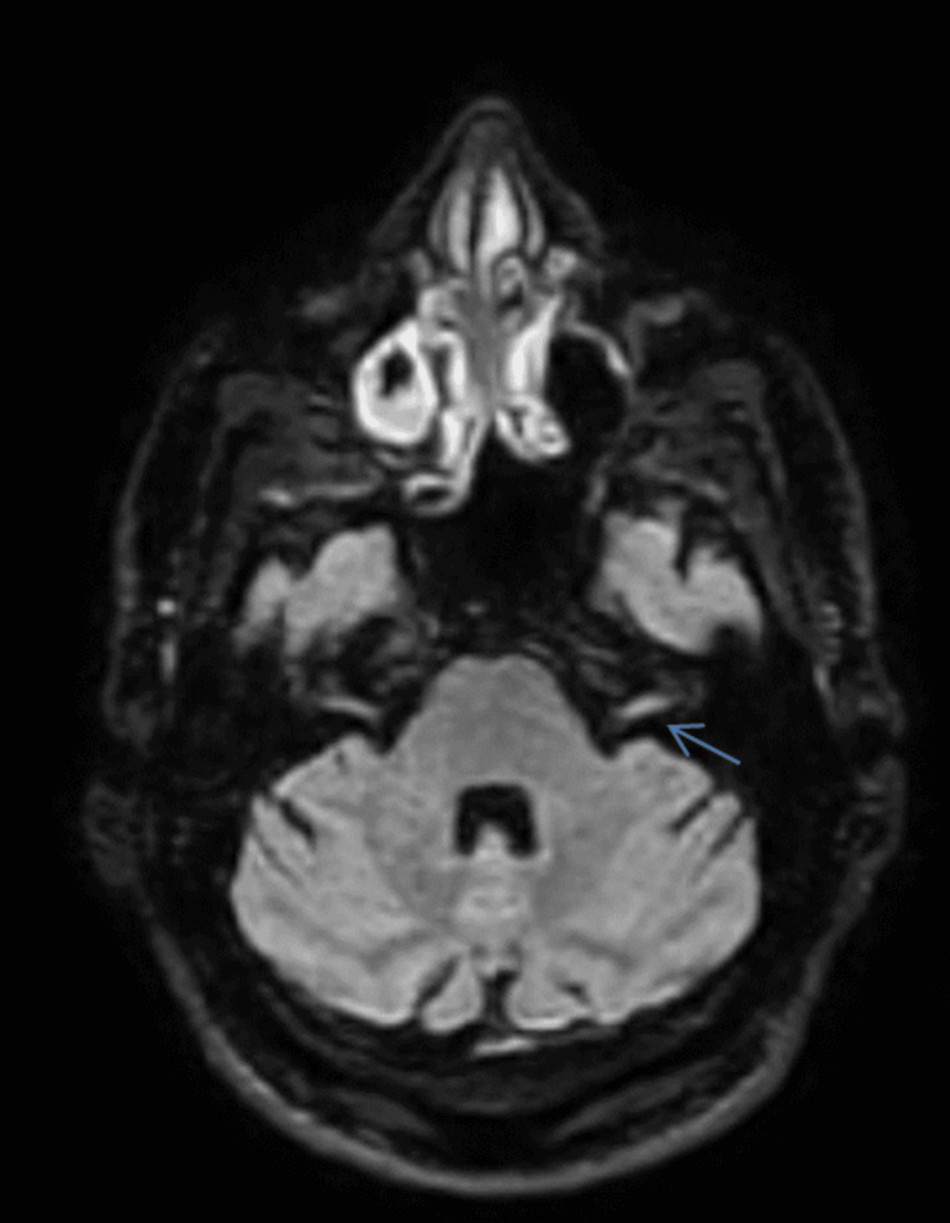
Axial T2-weighted FLAIR MRI of the brain demonstrating hyperintensity involving the left facial nerve (cranial nerve VII) in the cerebellopontine angle region (blue arrow). The increased signal intensity along the left facial nerve pathway is clearly visible compared with the normal-appearing right facial nerve. FLAIR: Fluid-Attenuated Inversion Recovery.
